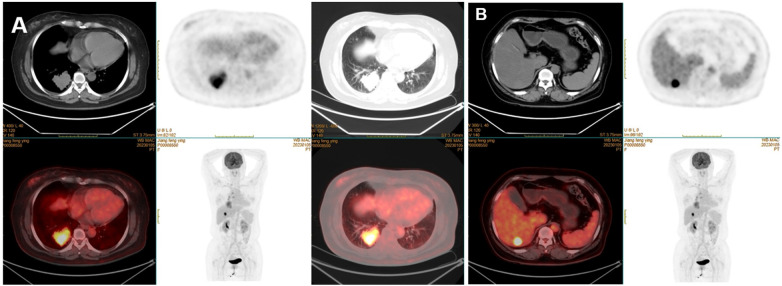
Representative ^18^F-FDG PET/CT images of a 54-year-old woman with EGFR L858R-positive lung adenocarcinoma (cT4N0M1c, stage ⅣB). (A) Primary tumor in the right lower lobe: SUV_max_=8.53, SUV_min_=3.34, SUV_avg_=4.77, SUV_peak_=7.52, TLG=240.46, MTV=50.41 cm^3^. (B) Hepatic metastasis: SUV_max_=13.66, SUV_min_=4.89, SUV_avg_=8.04, SUV_peak_=11.28, TLG=46.15, MTV=5.74 cm^3^.
